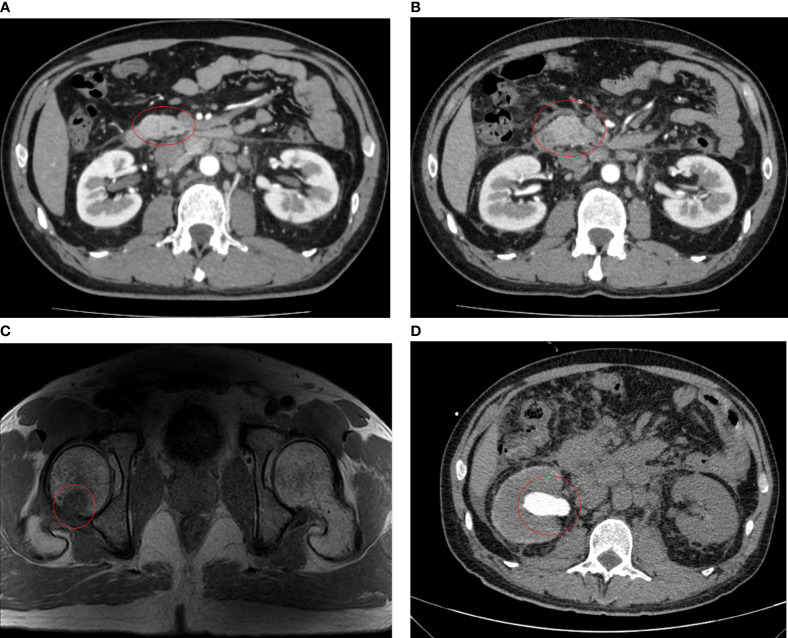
Imaging examinations performed after chemotherapy. (A) After five cycles of XELOX, CT scans on August 12, 2020 revealed enlargement of the abdominal para-aortic and bilateral para-iliac lymph nodes, about 0.5–1.5 cm, with slightly enhanced. (B) After six cycles of FOLFIRI plus cetuximab treatment, CT scans on November 28, 2020 revealed significant enlargement of lymph nodes in the hilar area, adjacent to the abdominal aorta, and bilateral iliac vessels, with a maximum of about 2.3 cm and slightly enhancement. (C) MRI scans on November 29, 2020 show patchy shadows are seen on the right femoral head and greater trochanter, enhanced scans are seen to be enhanced, consider metastasis. (D) CT scans on December 22, 2020 showed hydronephrodilation of the right renal pelvis and space-occupying lesion about 16.2 mm × 9.3 mm in the initial segment of the right ureter.
